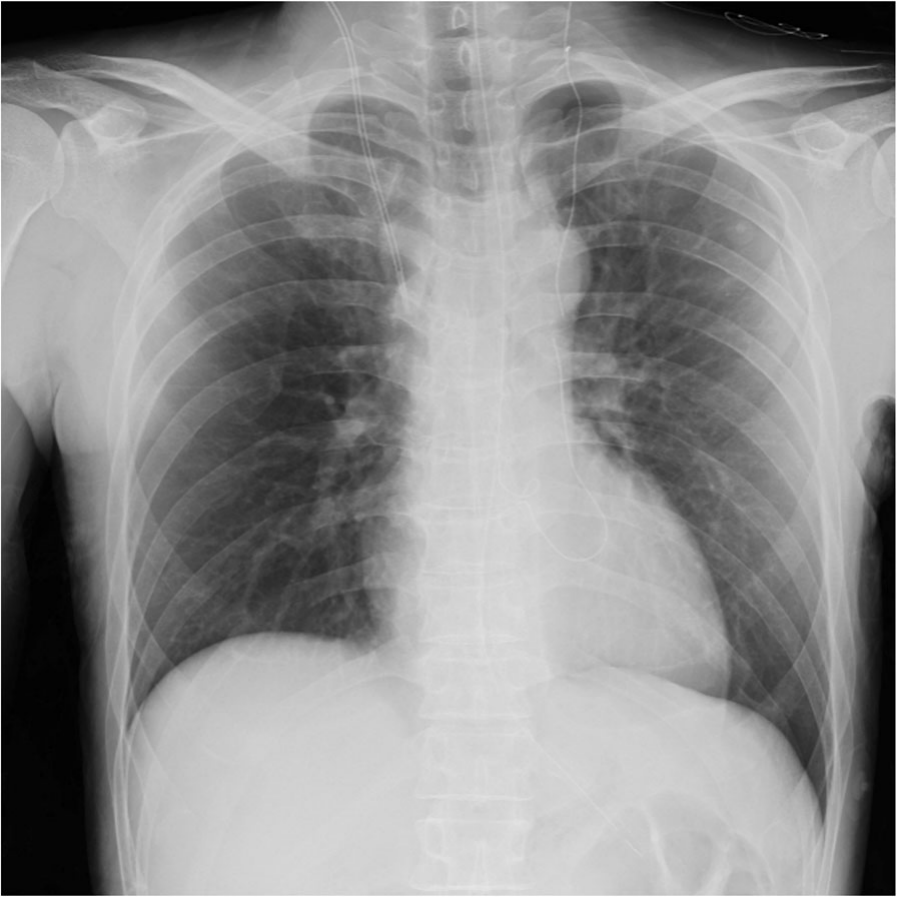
Chest X-ray 5 h after surgery showing a fully expanded left lung without re-expansion pulmonary edema
